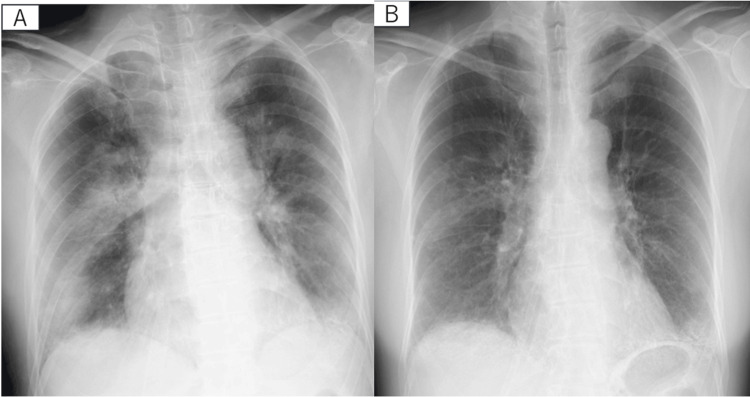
Chest X-ray one day after bronchoscopy (A) and two days after bronchoscopy (B).
